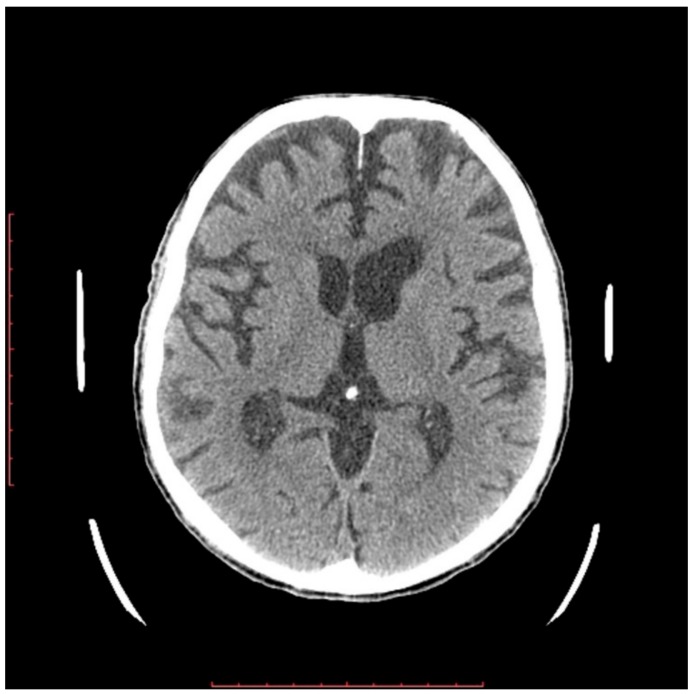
Computed tomography (CT) examination made before patient’s death. Progression of frontal lobe and subcortical atrophy with secondary ventricular dilatation are shown.
